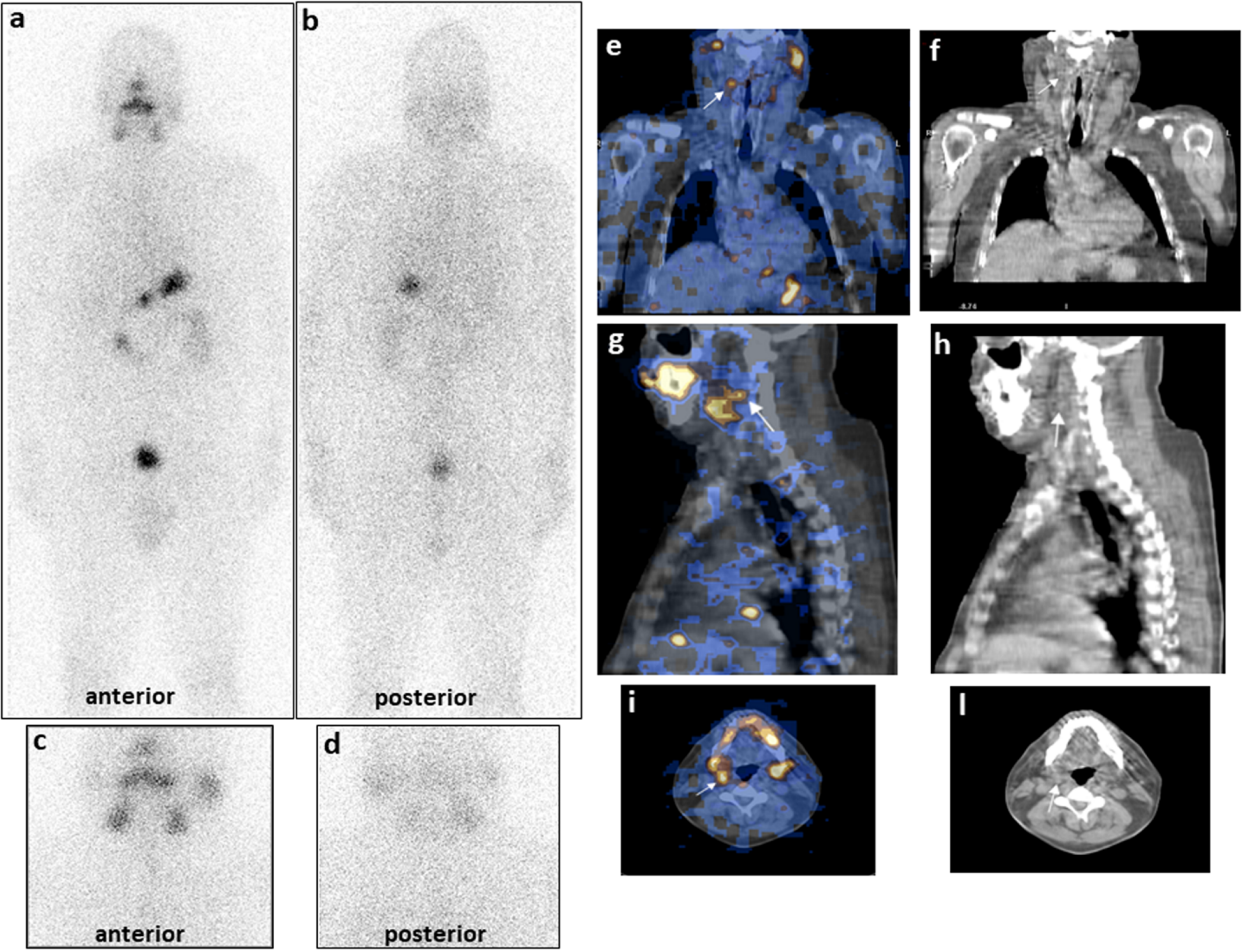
A patient in long-term follow-up affected by unifocal papillary carcinoma with extra-thyroid tumor extension (T4aN0M0) already submitted to total thyroidectomy and radioiodine ablation. Diagnostic planar 131I-WBS after rh-TSH stimulation, in both anterior and posterior views (a, b), and planar neck/thorax anterior and posterior views (c, d), do not show any foci of pathologic radioiodine uptake. SPECT/CT in coronal (e, f), sagittal (g, h) and transverse (i, l) fusion images evidenced one radioiodine-avid focus (white arrows) in right laterocervical region adjacent to the sub-mandibular gland, in a posterior position, classifying it as lymph node metastasis also visible at CT images. Thyroglobulin levels were undetectable in basal conditions reaching values of 7.8 ng/ml after rh-TSH stimulation; anti-thyroglobulin antibodies were negative. Thus, the patient underwent a second therapeutic dose of radioiodine
